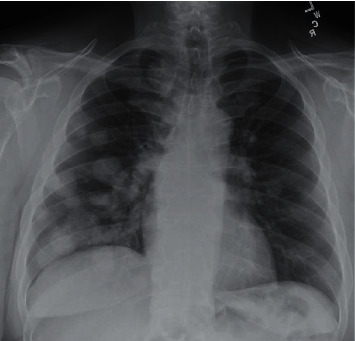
Initial chest X-ray showing multiple new pulmonary nodules.
